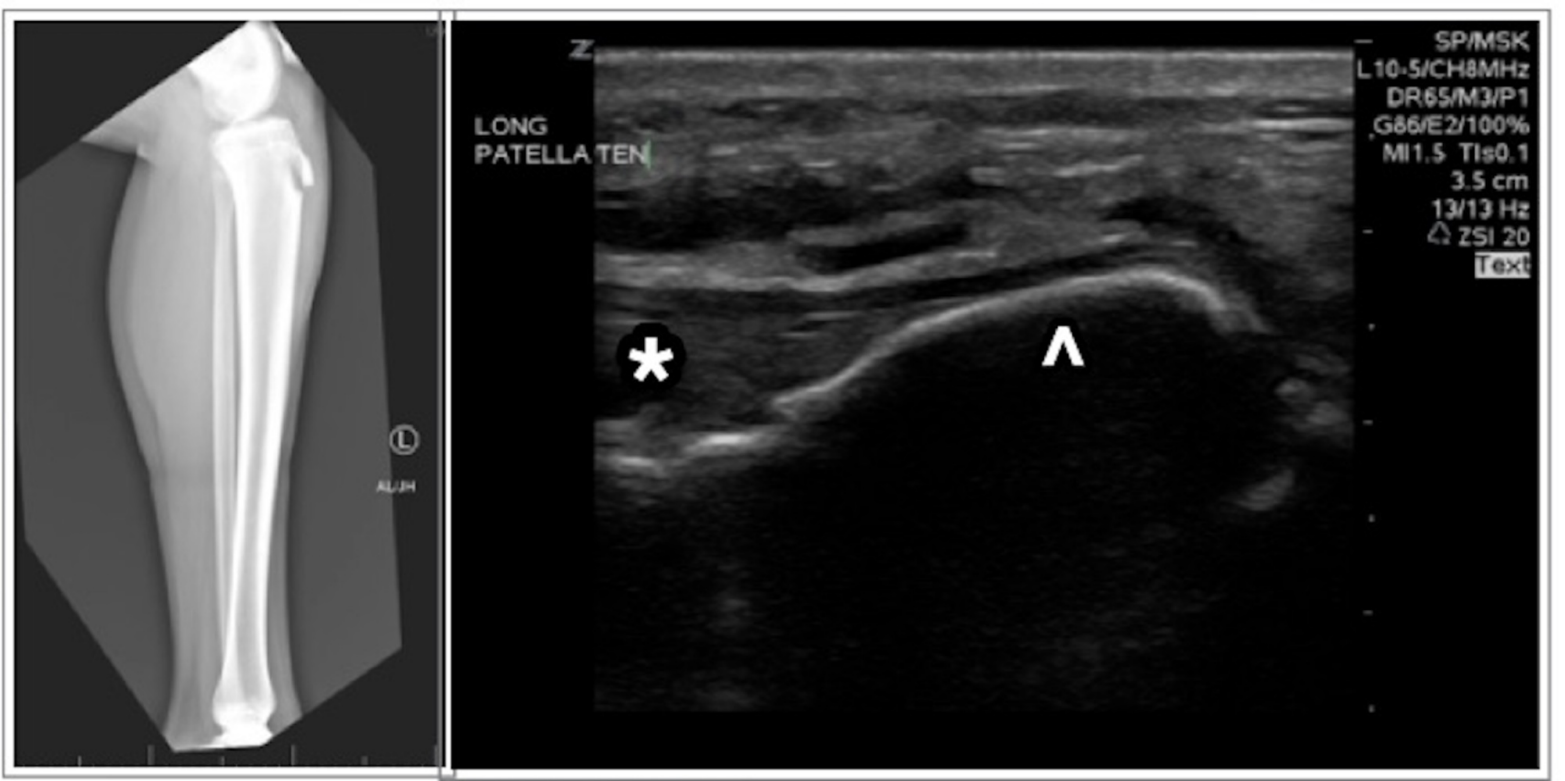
Radiograph demonstrating fracture of the left tibial tubercle (left); ultrasound of the knee (right) demonstrating avulsed tibial tuberosity (arrowhead) with attached patellar tendon (*)
